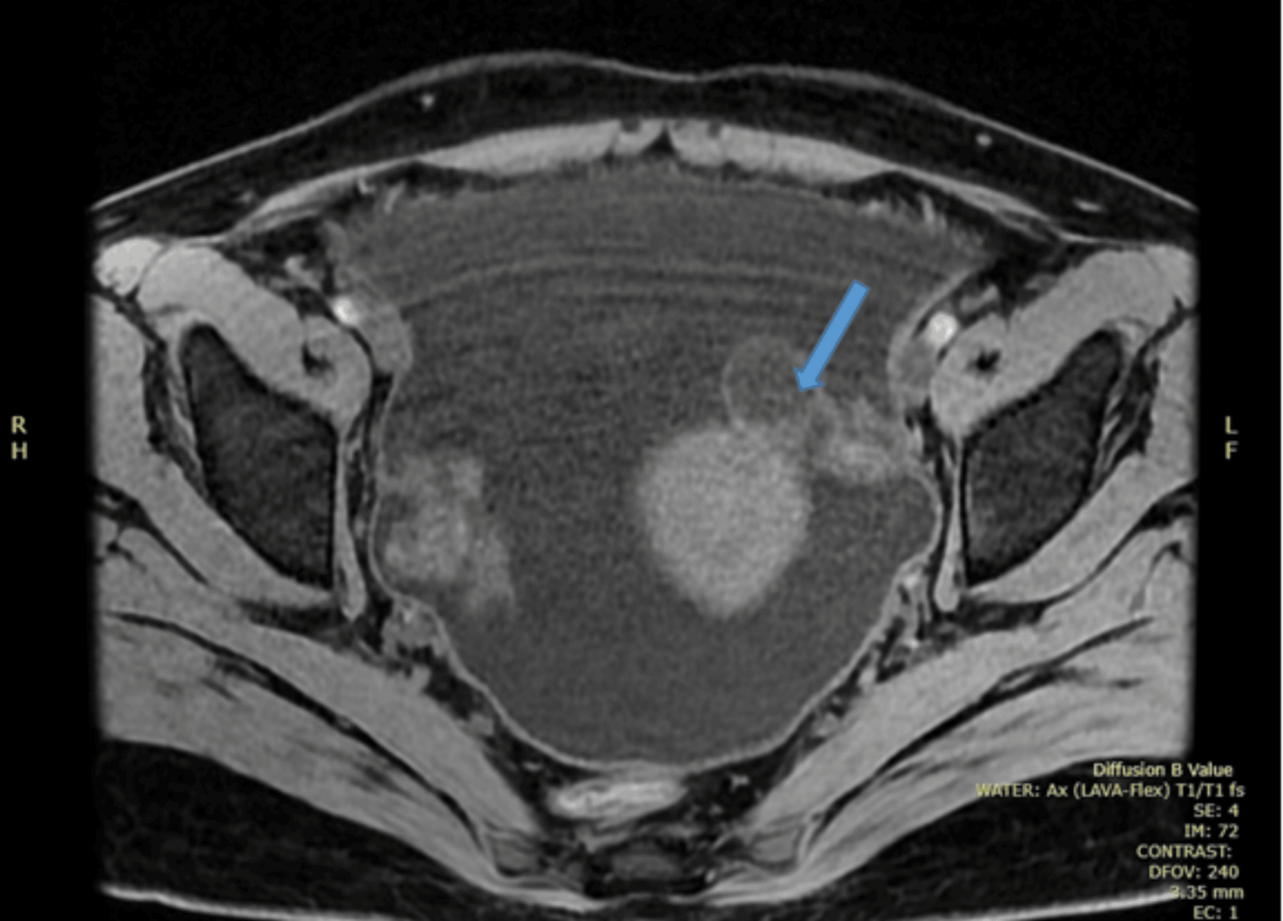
Initial MRI demonstrating a left ovarian cyst (blue arrow). MRI, magnetic resonance imaging
